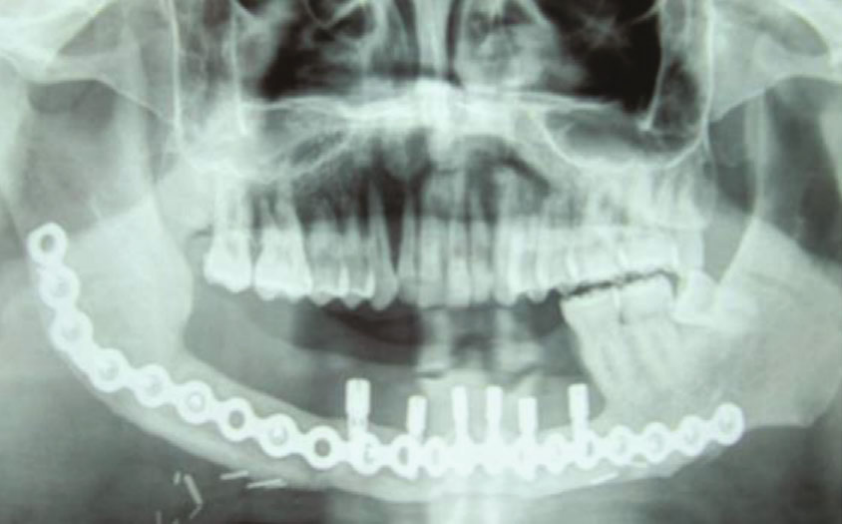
Orthopantomography after 6-implant placement on the fibula.
